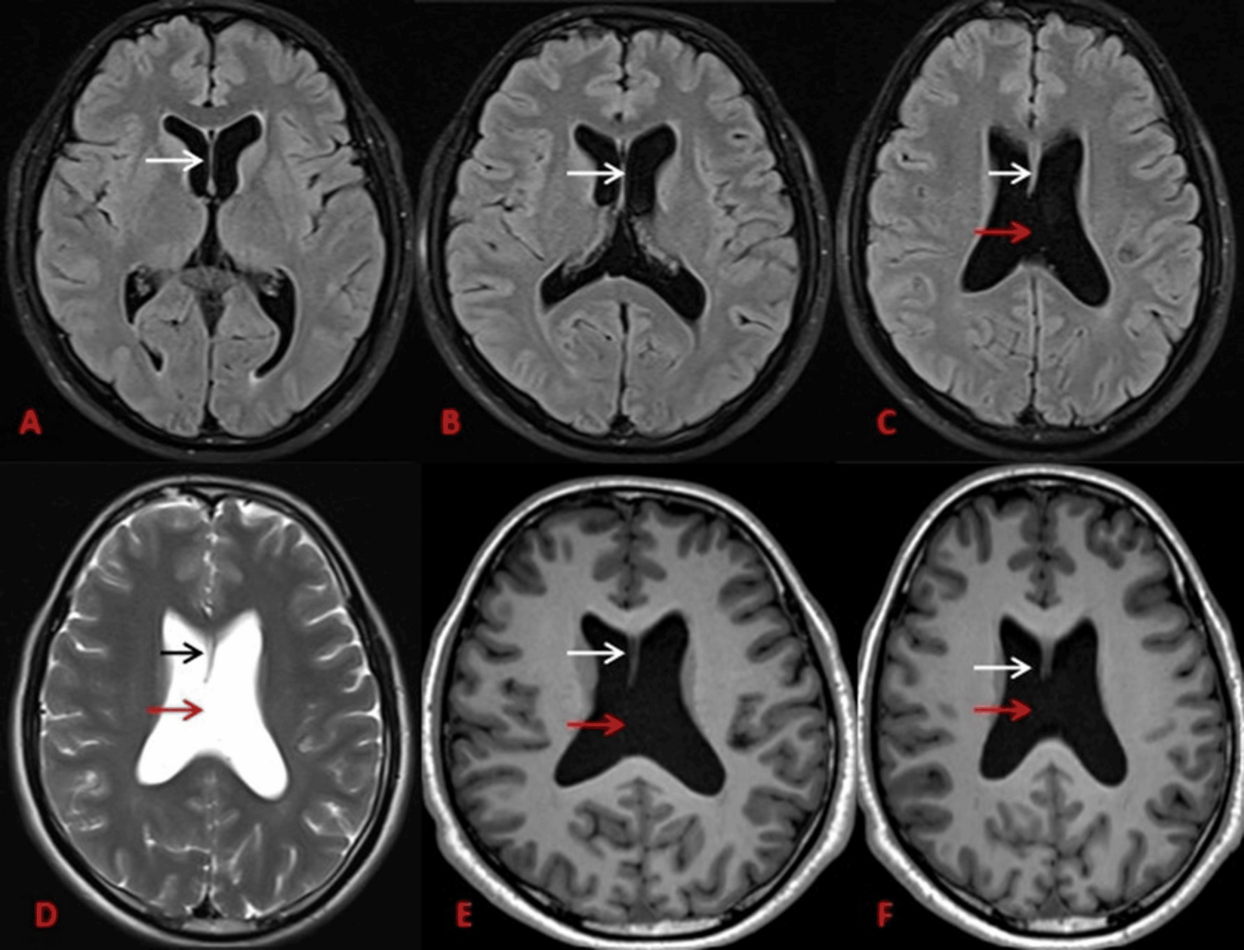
MRI brain (Axial): A-F showing the anterior portion of the septum pellucidum in A-C-FLAIR, D-T2WI, and E-F-T1WI (marked by black and white arrows) and absence of the posterior portion of the septum pellucidum (C-F) (marked by the red arrow). FLAIR: fluid-attenuated inversion recovery, T2WI: T2-weighted imaging, T1WI: T1-weighted imaging
